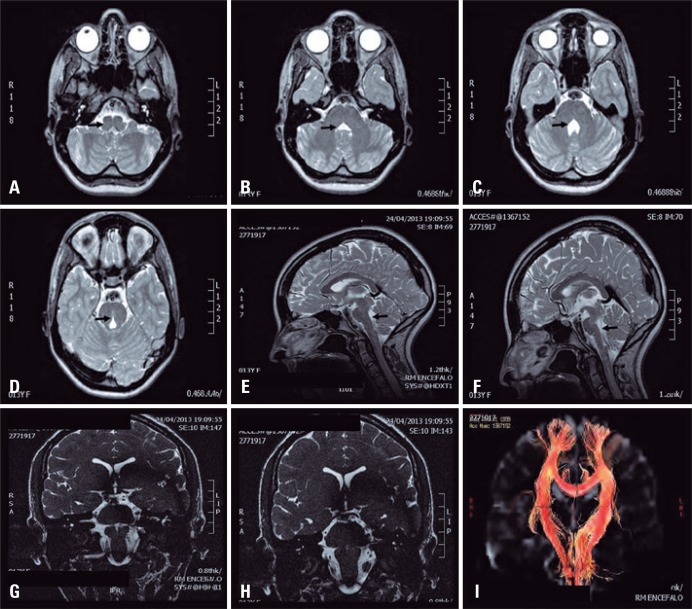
Pre-operative images. (A to D) Magnetic resonance imaging axial T2 shows the hypoplastic pons and medulla with a prominent midline cleft. (E and F) Magnetic resonance imaging sagittal T2 of the brain shows depression of the floor of the fourth ventricle. The pons and medulla oblongata have a reduced volume. (G and H) Magnetic resonance imaging coronal T2. (I) Tractography showed no crossing over of major fibers at the level of pons
